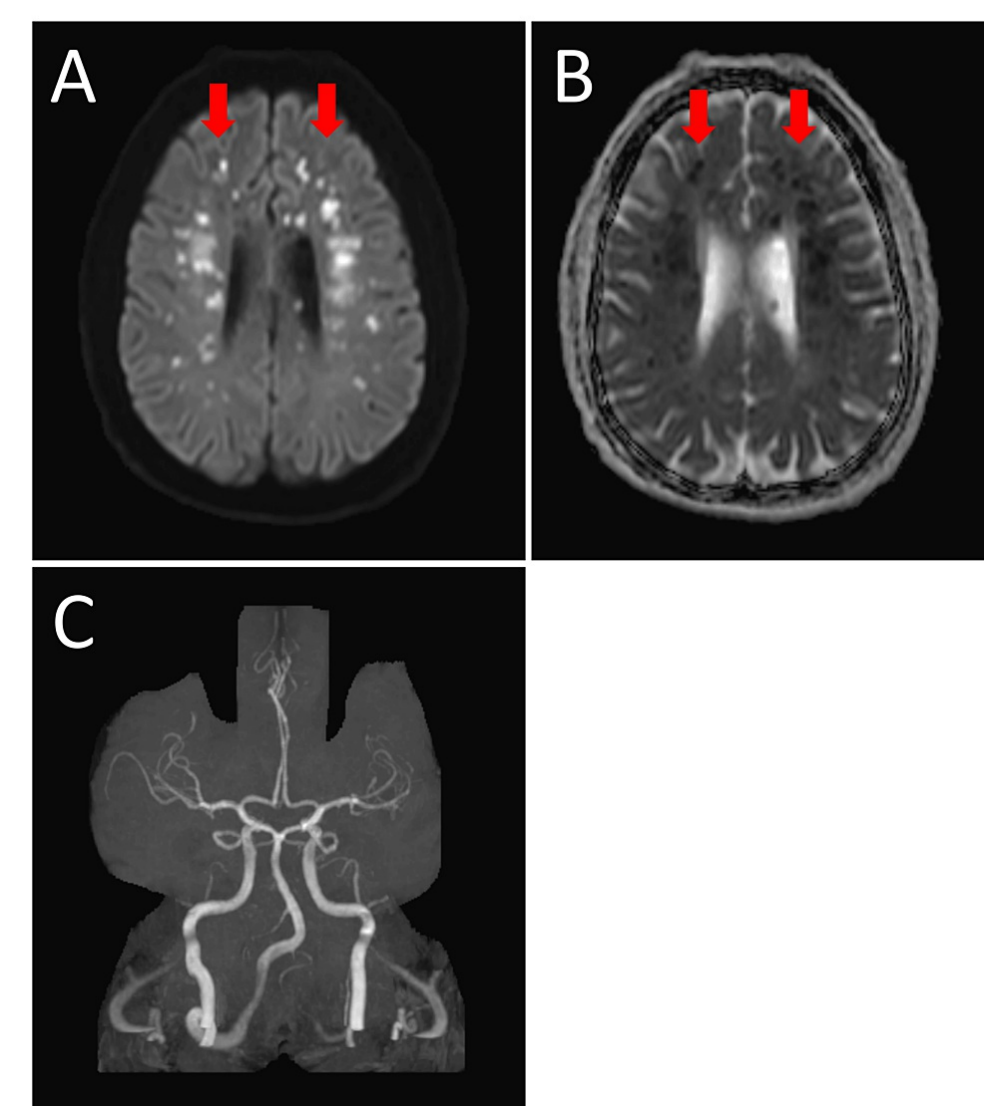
MRI head without contrast demonstrating multiple foci of infarction indicated by red arrows and MRA of the circle of Willis showing no evidence of large vessel occlusion. 2A: Axial view of diffusion-weighted imaging (DWI) MRI; 2B: Axial view of T2-weighted MRI; 2C: 3-D MRA. MRA: Magnetic resonance angiography.
